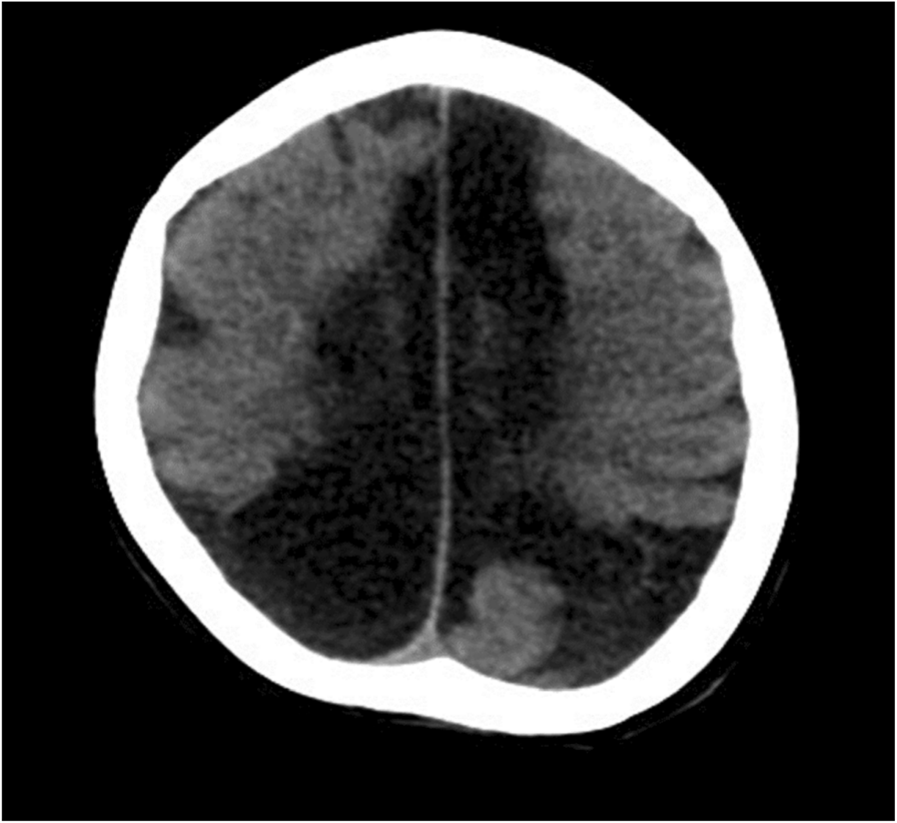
Postnatal head computed tomography scan of second baby at 6 years old showing hypodense areas over parasagittal regions of bilateral cerebral hemispheres, in keeping with multicystic encephalomalacia related to perinatal insults
